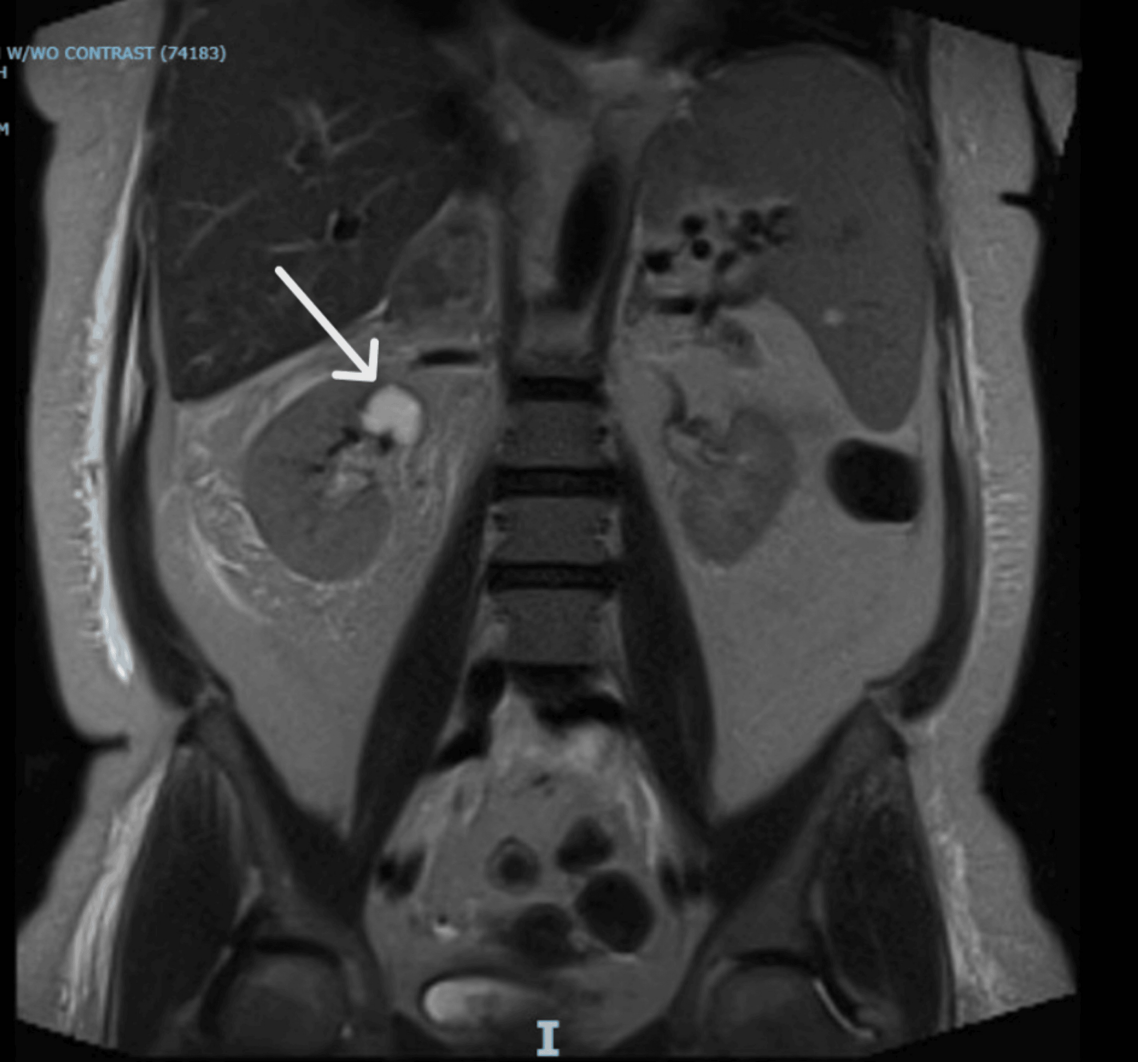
MRI abdomen without contrast. A well-defined mass within the right adrenal gland, which is hyperintense on T1-weighted images, is consistent with a hematoma (arrow).
